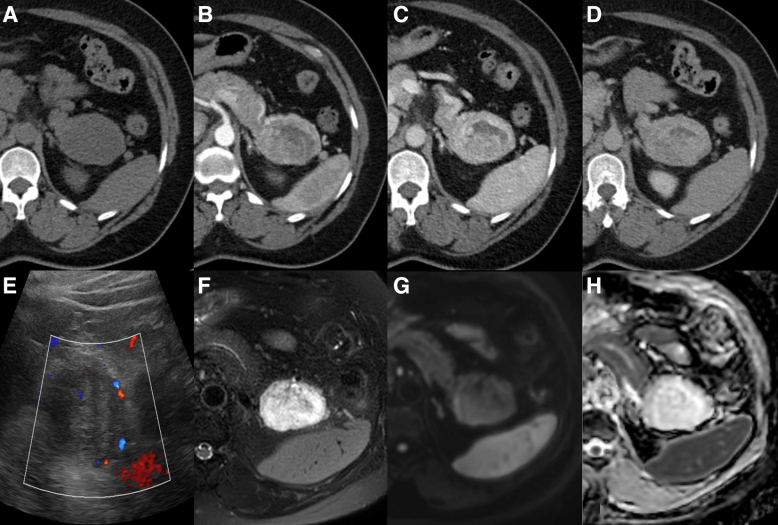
Multimodality imaging findings of a solid serous adenoma in the pancreatic tail. (A) Precontrast CT image shows lower attenuation of the lesion than of the adjacent pancreatic parenchyma. (B) Arterial-phase CT demonstrates heterogeneous peripheral enhancement. (C) Portal venous-phase CT shows progressive centripetal fill-in. (D) Delayed-phase CT image shows gradual enhancement of the central portion, whereas a crescent-shaped region remains persistently hypoattenuating. (E) Transabdominal ultrasonography demonstrates a well-defined ovoid mass with heterogeneous echogenicity and mild peripheral vascularity on color Doppler imaging. (F) T2-weighted MR image shows heterogeneous hyperintensity. (G) Diffusion-weighted image shows mildly increased signal intensity. (H) Apparent diffusion coefficient map shows no corresponding low signal intensity, indicating the absence of true diffusion restriction. CT = computed tomography, MR = magnetic resonance.
